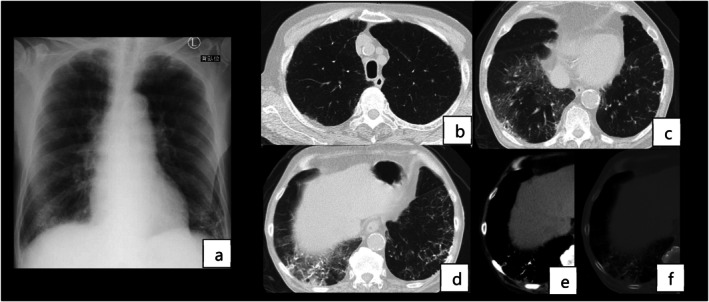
Chest radiography and computed tomography images on admission.
